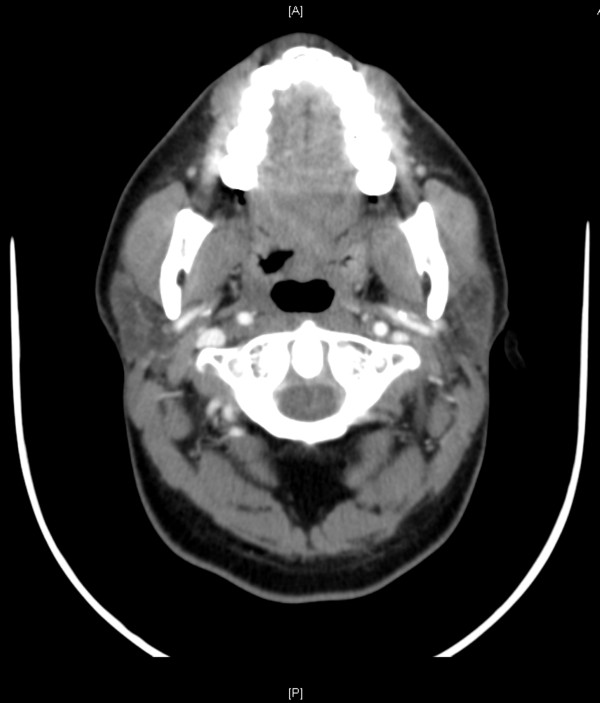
Computed tomography scan of the head and neck (cross-section) after chemoradiation showing resolution of the lesion.
